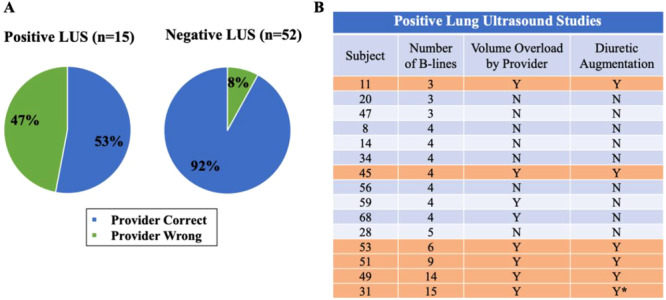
Provider assessment of volume status versus LUS. (A) Agreement of provider assessment of volume status with LUS. Providers' assessments were correct 92% of the time when the study was negative, but they only identified volume overload in half of the subjects with a positive LUS. B. Table showing details of the 15 subjects with positive LUS by ABLC. Number of B‐lines, provider assessment of volume status, and whether diuretics were augmented are shown. Orange‐shaded rows indicate diuretic augmentation. *Denotes the only subject admitted to hospital for intra‐venous diuretics. All subjects who had six or more B‐lines required diuretic augmentation; only the subject with the highest B‐line count required admission for intra‐venous diuretics. ABLC = automated B‐line counter, LUS = lung ultrasound, N = no, Y = yes.
